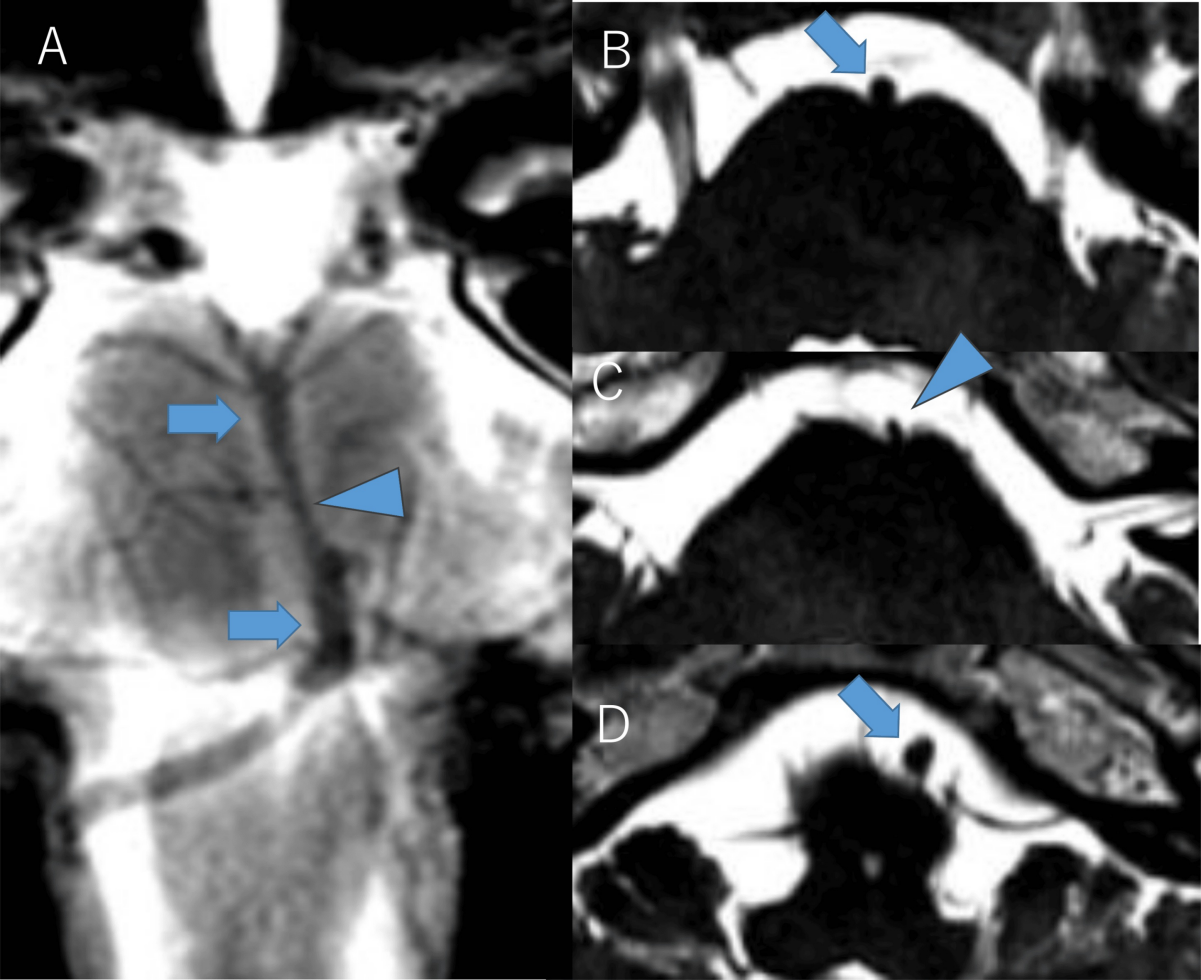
MRI showing shrinkage of the outer diameter in basilar artery (BA) of case 2. Basi-parallel anatomical scanning (A) and heavy T2-weighted images (B-D) acquired to evaluate the outer diameter of BA revealed that the outer diameter of the stenosed portion of BA was smaller than the non-stenosed portion. The outer diameters of the proximal part, stenosed part, and the distal part of BA were measured as 3.0 mm (B, blue arrow), 1.9 mm (C, blue arrowhead), and 3.8 mm (D, blue arrow), respectively, which highlighted the shrinkage of the outer diameter of the stenosed portion.
